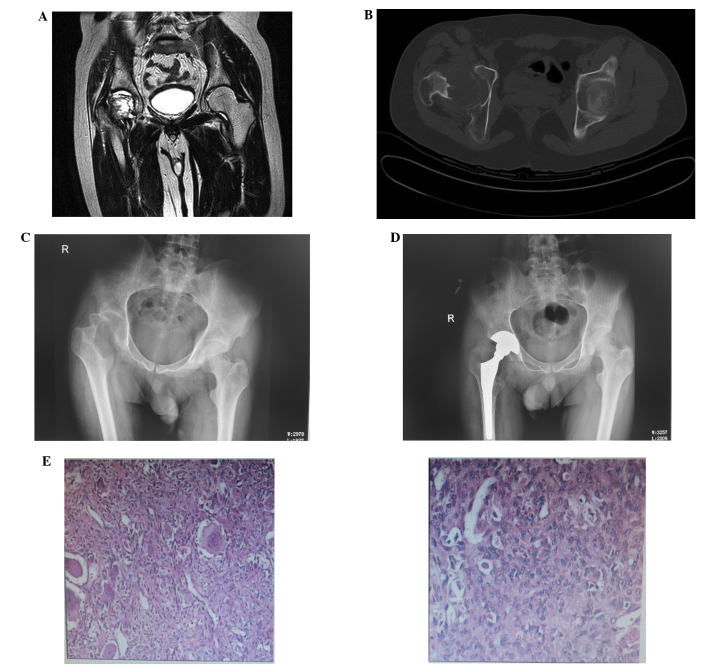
A 26-year-old male complained of pain right hip and claudication for 1 month. (A) Preoperative magnetic imaging revealing right proximal femur bone giant cell tumors. (B) Preoperative computed tomography scan showing a radiolucent, expansile, lytic lesion in the right femur head bone. (C) Preoperative radiographs showing right femur head giant cell tumors, pathological fracture and dislocation of the hip. (D) Five years following tumor resection using the ultrasonic scalpel, X-ray imaging indicates that total hip replacement and prosthesis position is good. (E) Pathological examination of resected tissue indicates cystic and necrotic tissue.
